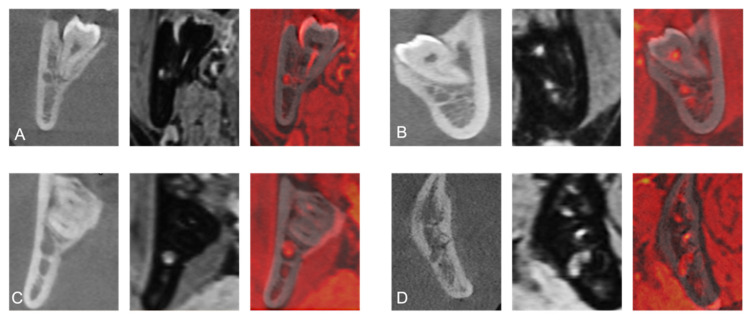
Coronal reconstruction of a study participant’s CBCT, 3D-DESS MRI, and fused CBCT/MRI images visualizing periradicular (buccal (A); lingual (B); inferior (C)) or interradicular (between the roots (D)) relative positional relationship of the IAN at the third molar.
